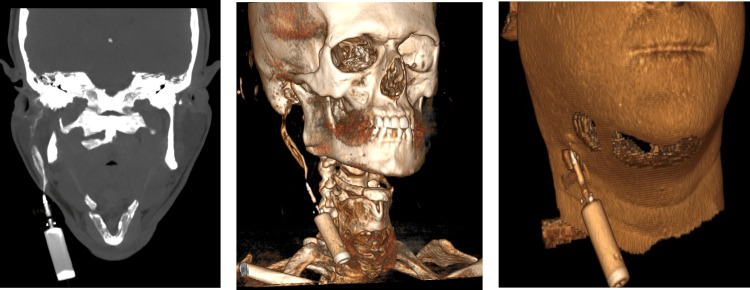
CT fistulography The green arrows depict the course of the fistula with a maximum diameter of 1.6 cm, starting from the external auditory canal, continuing through the parotid tissue, and ending in the right submandibular skin region (pane a shows the coronal view); (b and c) 3D rendering images show again the course of the fistula, demonstrating hard and soft tissue structures, respectively.
